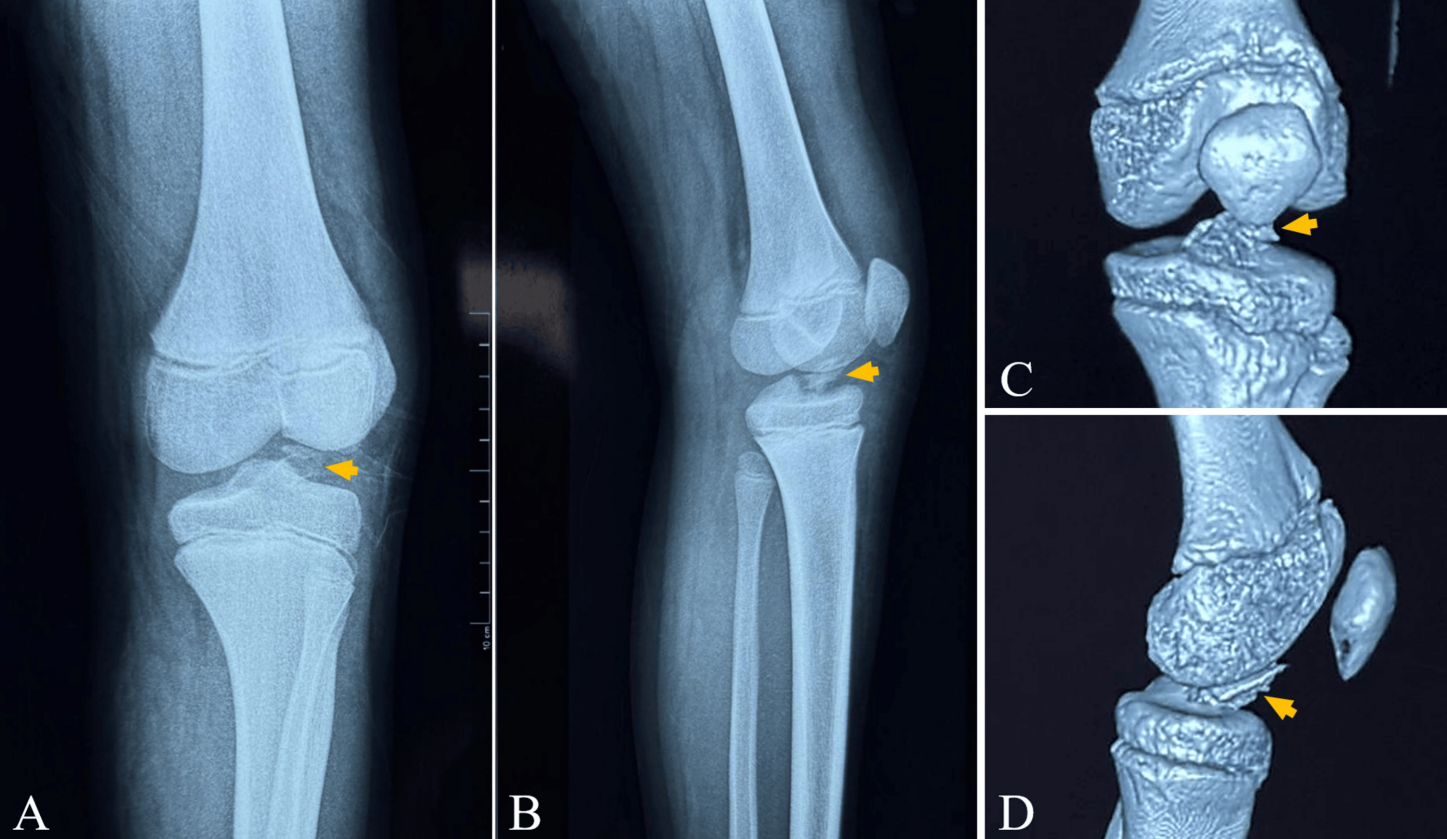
Plain radiograph and CT findings Initial plain radiographs show a displaced tibial spine fracture on the anteroposterior (A) and lateral (B) views. Three-dimensional CT reconstructions demonstrate the avulsed fragment in en face (C) and lateral (D) views CT: computed tomography
